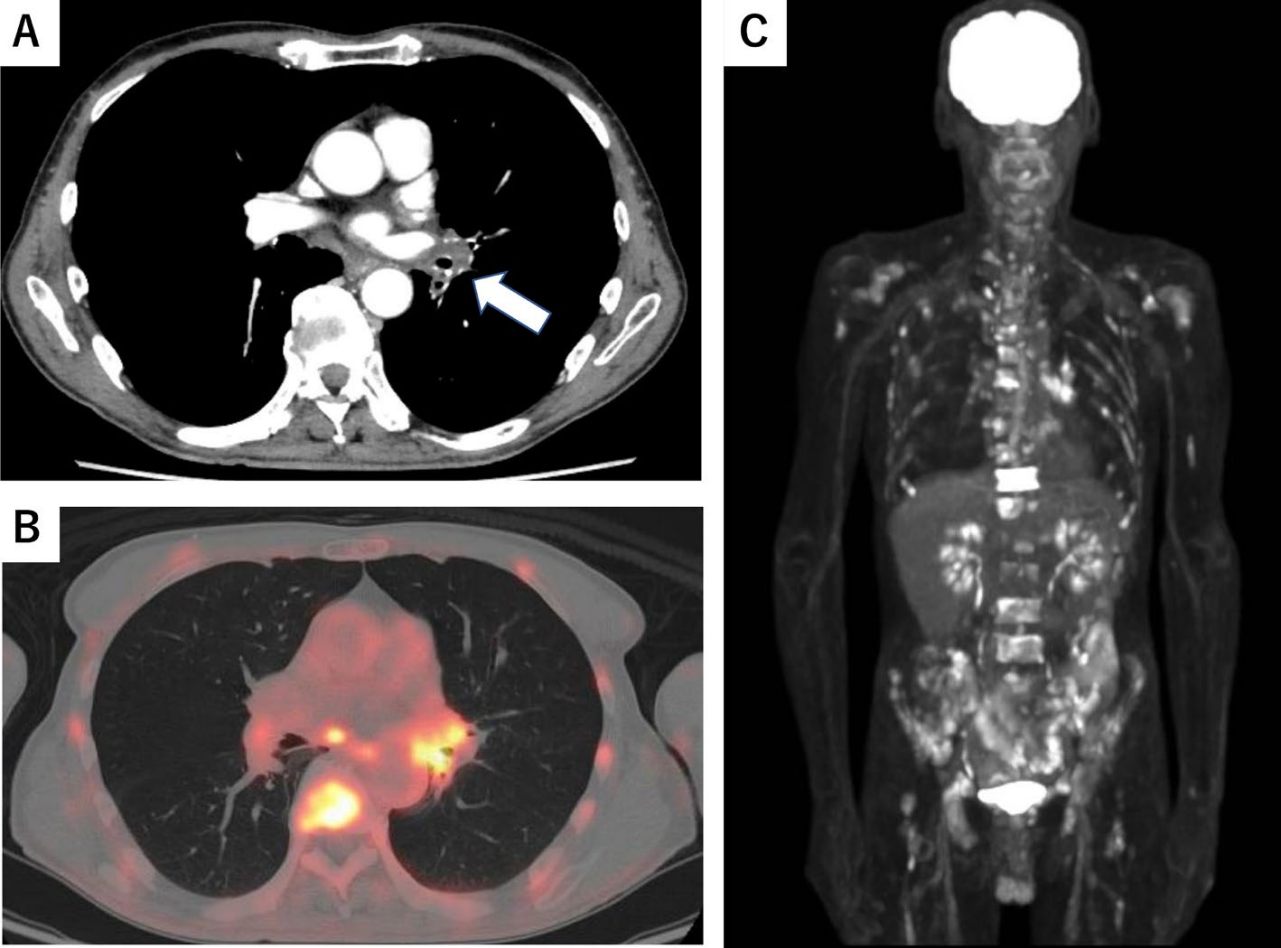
Radiographic findings at initial diagnosis and before immunochemotherapy. (A) Chest computed tomography revealed left main bronchial wall thickening and left hilar lymph node swelling. (B, C) Positron emission tomography with fluorodeoxyglucose‐computed tomography (FDG‐PET/CT) showed uptake in left hilar lymph node and multiple bone lesions, including the spine and the bilateral humerus and femur.
